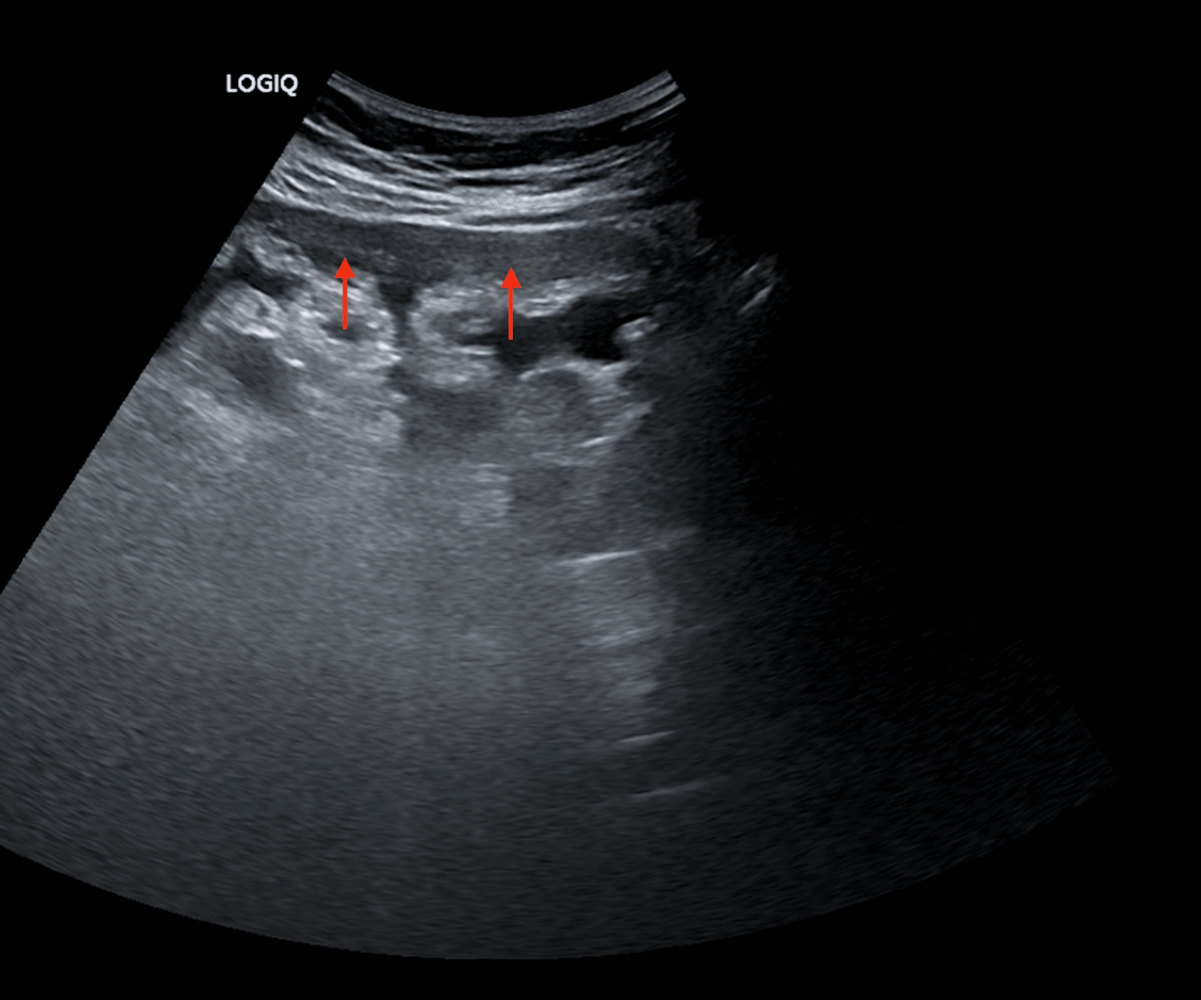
Abdominal ultrasonography showing omental thickening Ultrasound image showing omental thickening, or omental 'caking', indicated by the red arrows, which is suggestive of pathologic involvement of the omentum.
